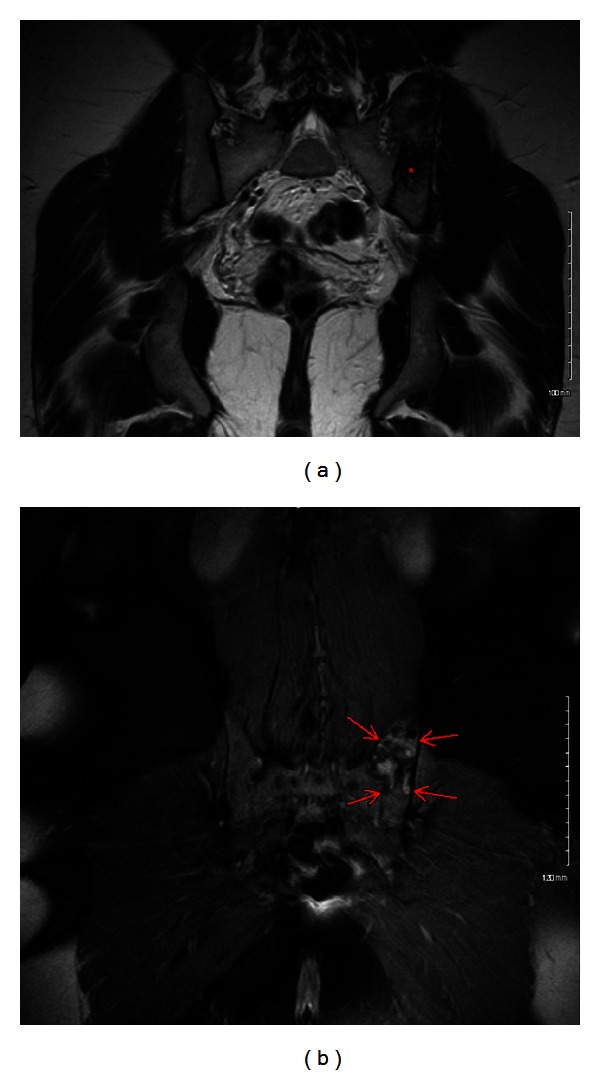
(a) Coronal T2-weighted MR image with gadolinium contrast. Lytic area within the bone lesion is noted with red asterisk. (b) Coronal T2 fat-saturated MR image without contrast. Mixed lytic/sclerotic lesion (red arrows).
